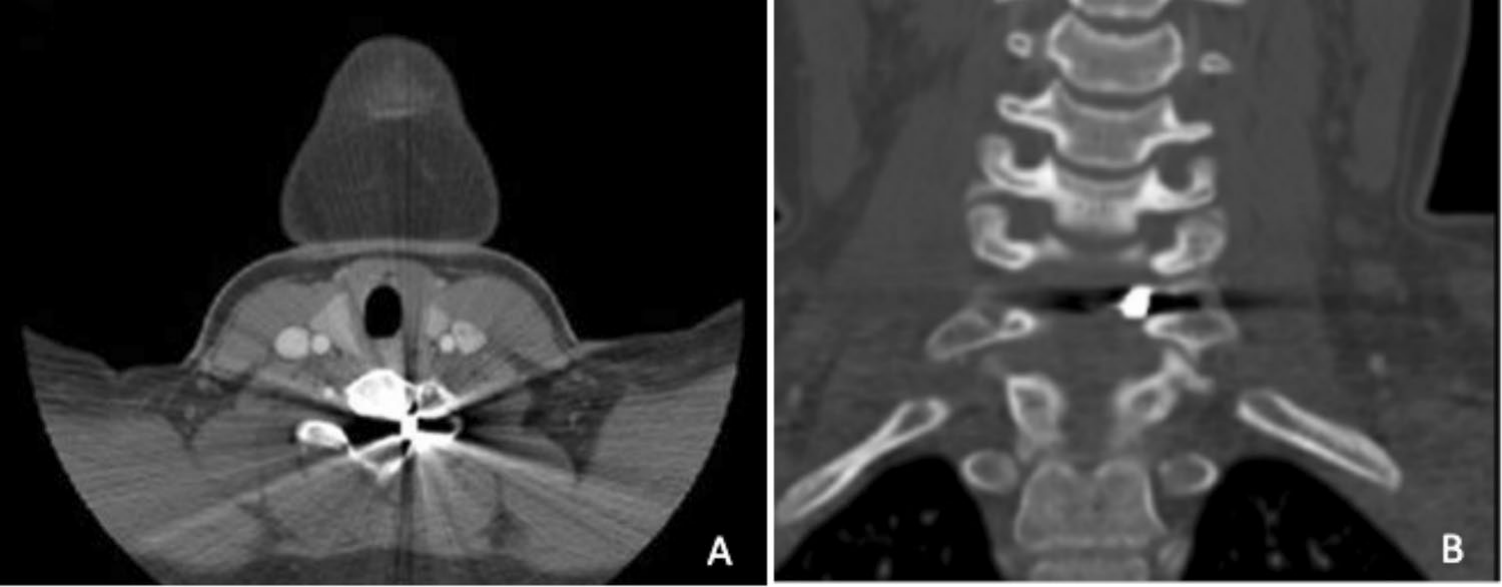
Preoperative CTA confirming the location of the pellet. A Axial view. B Coronal view
